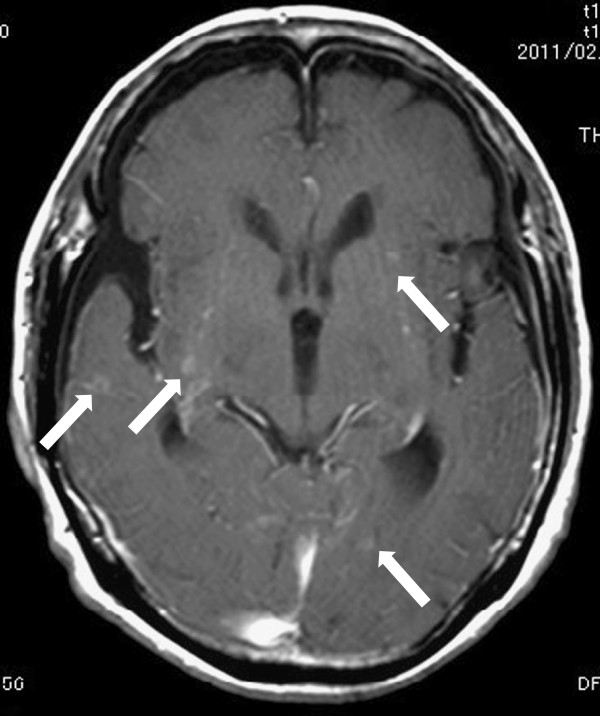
T1-weighted gadolinium contrast-enhanced magnetic resonance imaging showed multiple enhanced tiny nodules (arrows).
